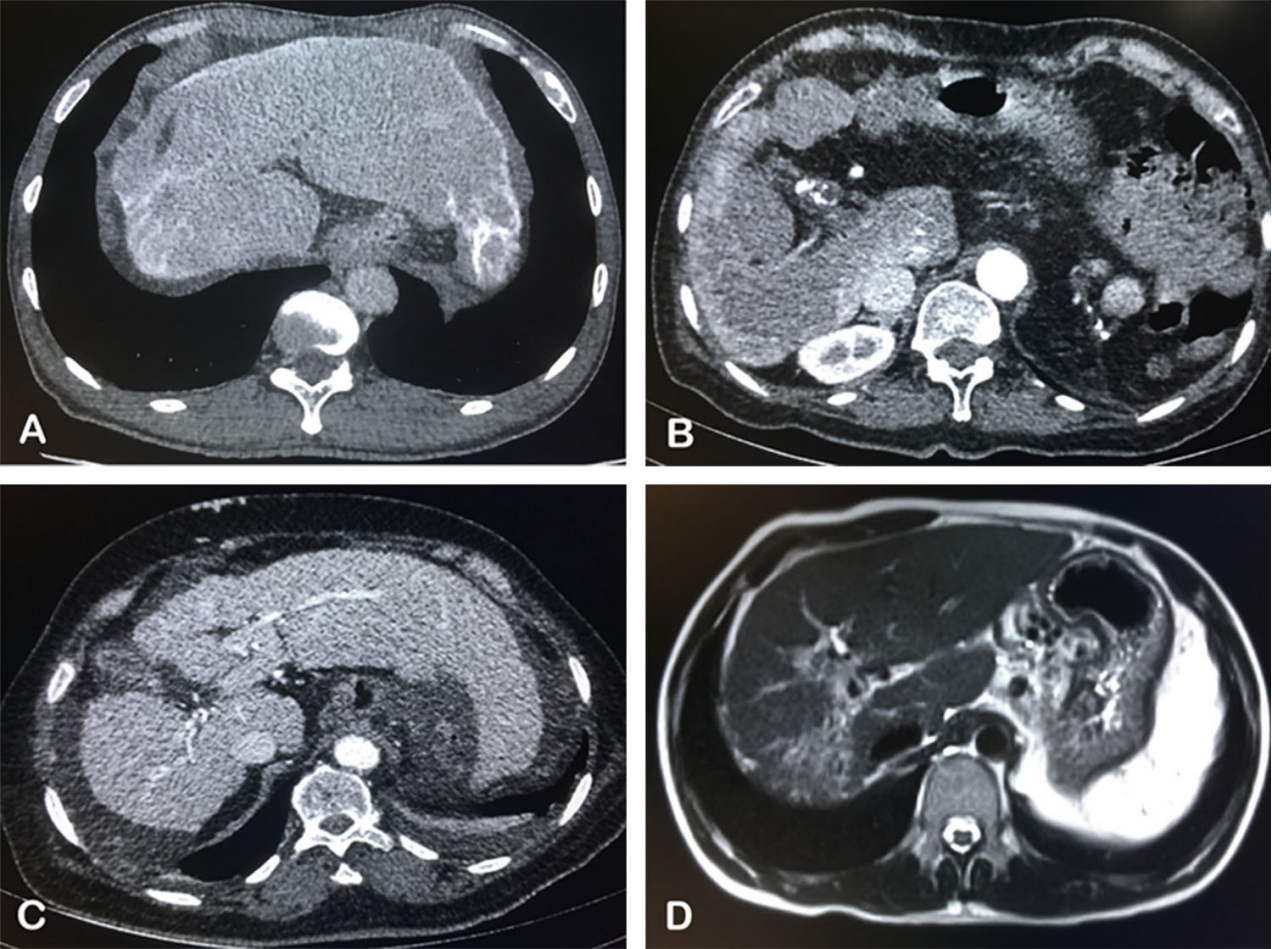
Typical symptoms of schistosomiasis-induced liver fibrosis. A The capsular calcification was found in the CT scan of a 52-year-old man; B widening of ligamentum teres, portal vein thrombosis (PVT) and insufficient liver perfusion in the CT enhanced scan of a 54-year-old woman; C ascites and prominent collateral circulation in the CT enhanced scan of a 70-year-old woman; D portal branches fibrosis in the T2-weighted image of a 31-year-old man
